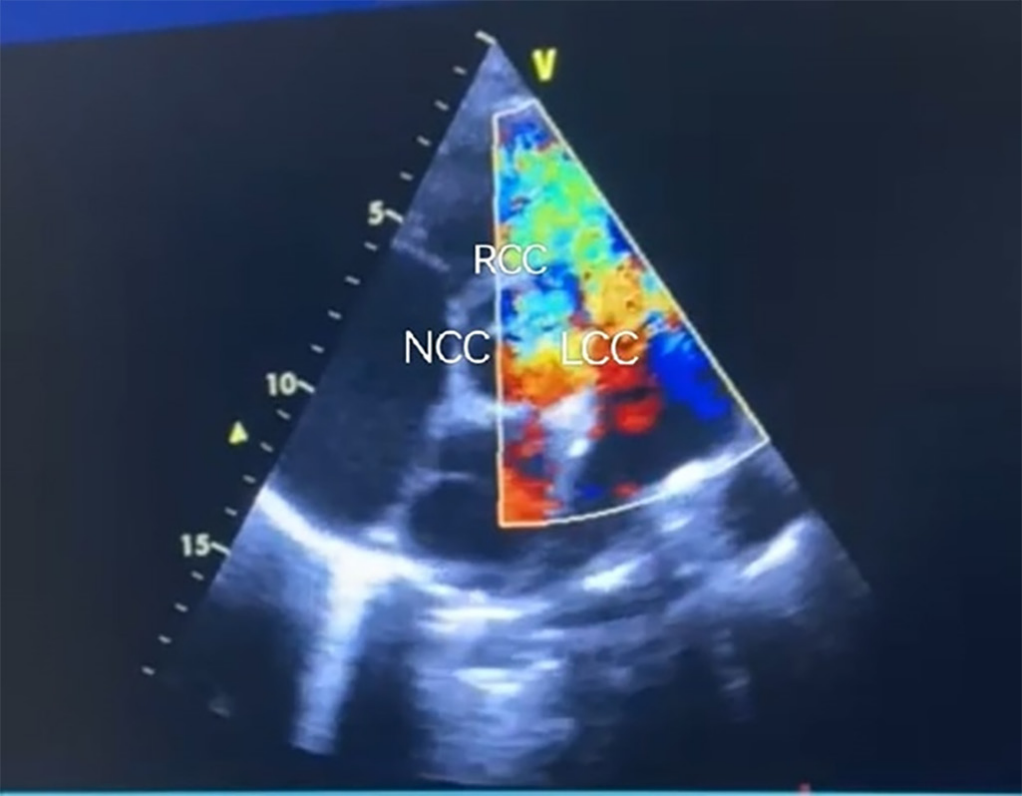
Transthoracic echocardiography. The arrow shows a ruptured sinus of Valsalva of the right coronary cusp leading to the left to right shunt.
